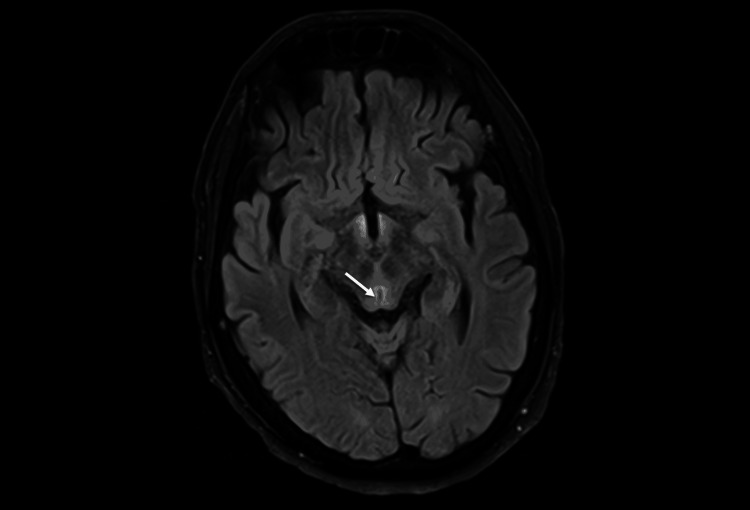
Axial T2 FLAIR, periaquedutal grey matter hyperintensity FLAIR: fluid-attenuated inversion recovery
